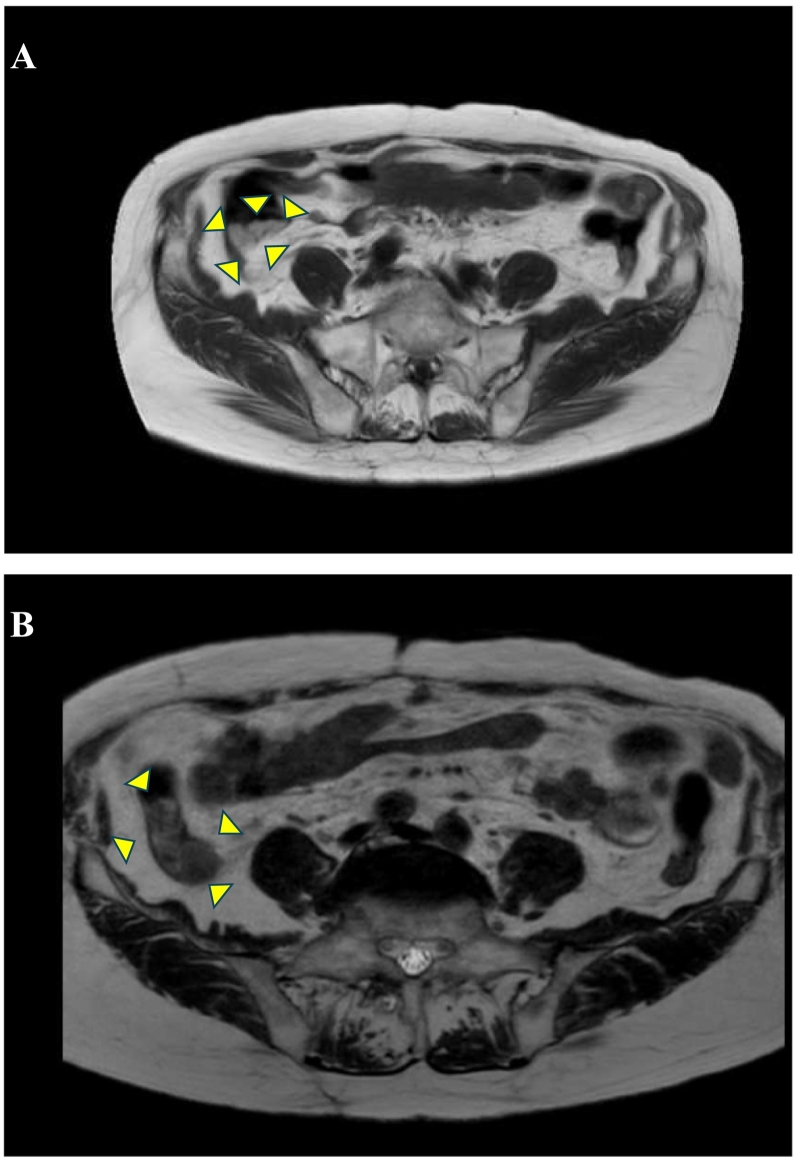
Magnetic resonance imaging (MRI) findings. MRI showed a pale high signal on T2-weighted and diffusion-weighted images, as well as a high signal on fat-suppressed T1-weighted images, suggesting mucus accumulation.
